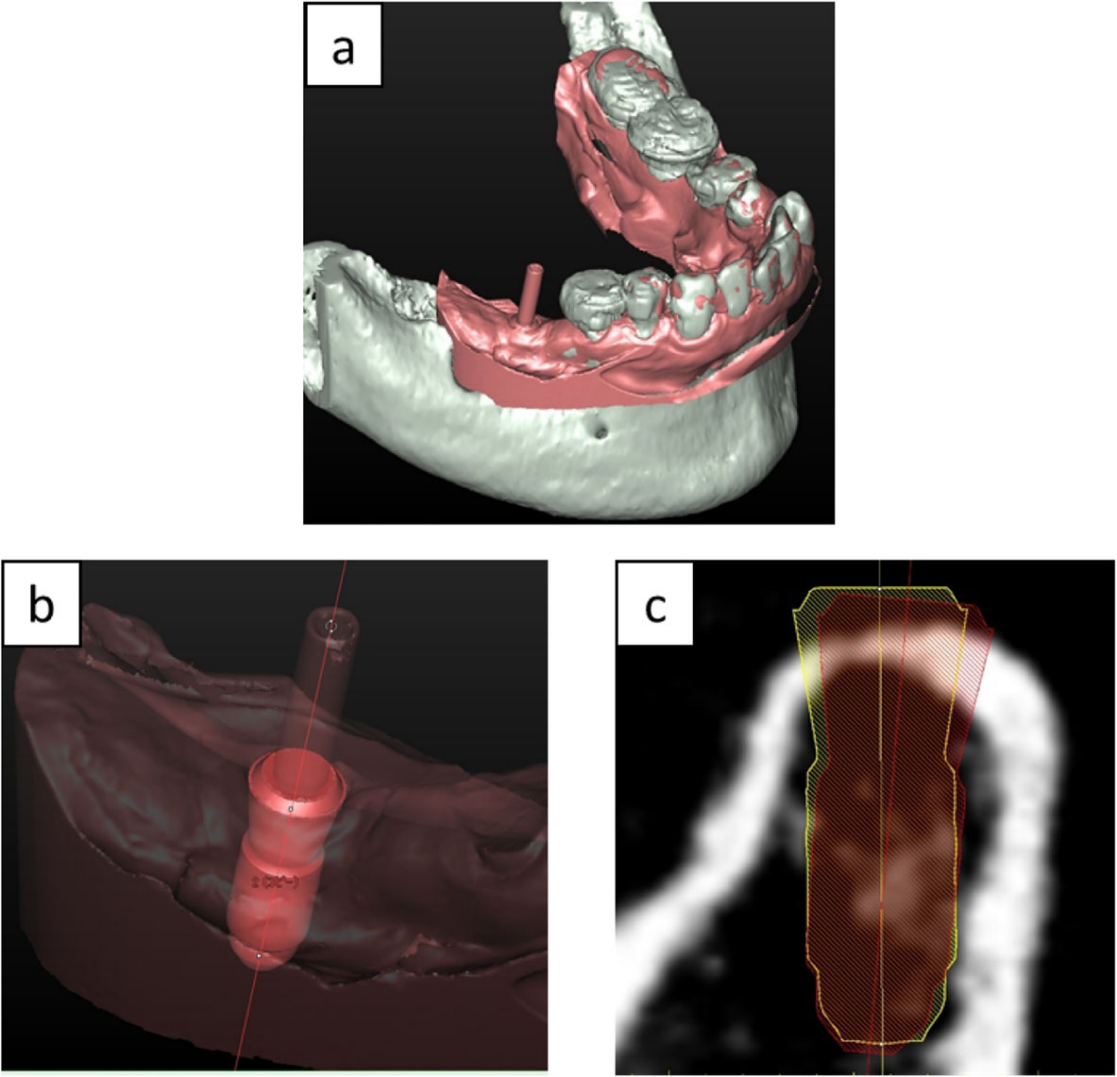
Assessment of placed implant position. a Matching digital data between preoperative planned computed tomography image (gray-colored model) and scanned postoperative impression (pink-colored model). b Assessing placed implant position using implant collar and guide pin as references. c Comparing planned (yellow-colored) and placed (red-colored) implant position
